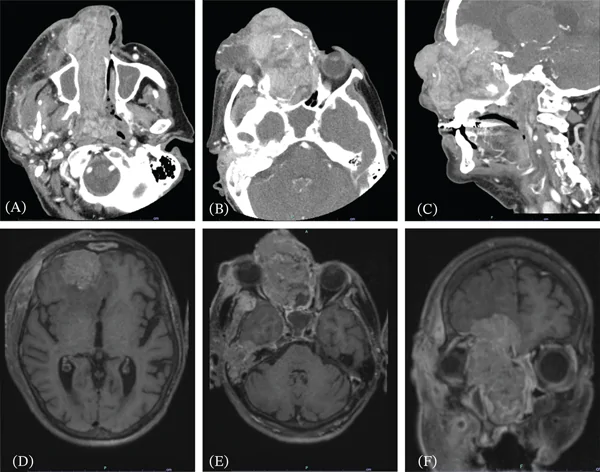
CT (A–C) and MRI scans (D–F) demonstrate a large nasal mass with intracranial and orbital involvement.
